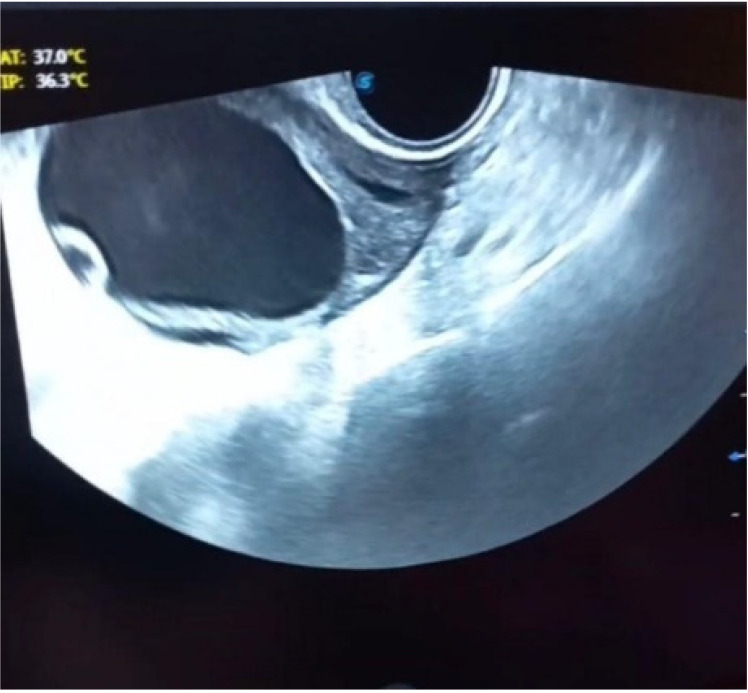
Cystic mass with a lobular margin shown by abdominopelvic sonography
